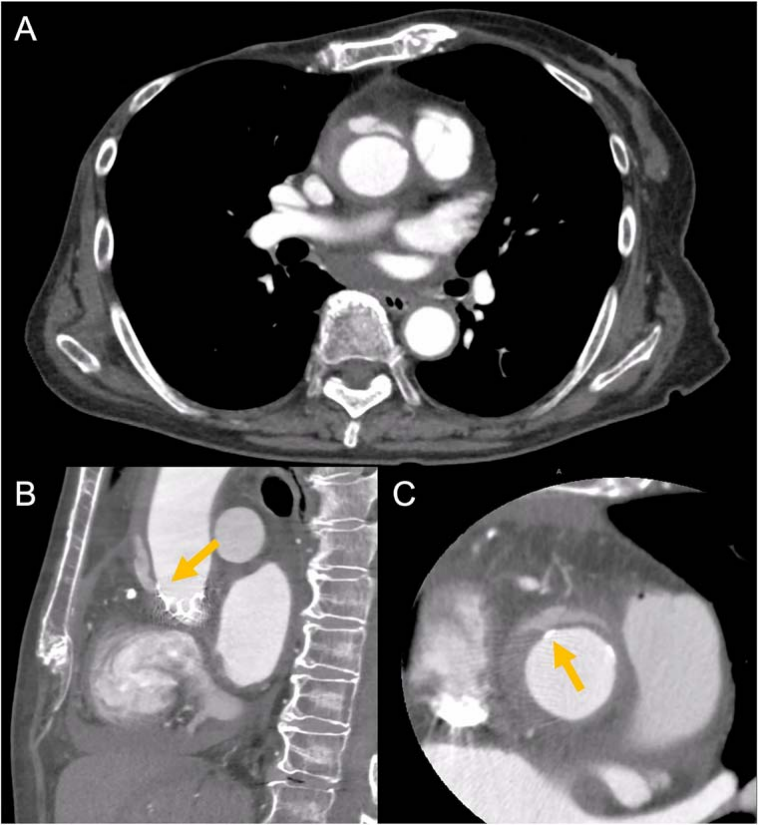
Contrast-enhanced CT on day 7 after discharge shows an AAD in the ascending aorta (A) with the entry point at the STJ extending toward the THV stent frame, suggesting an AAD associated with TAVI (B and C, arrow).
